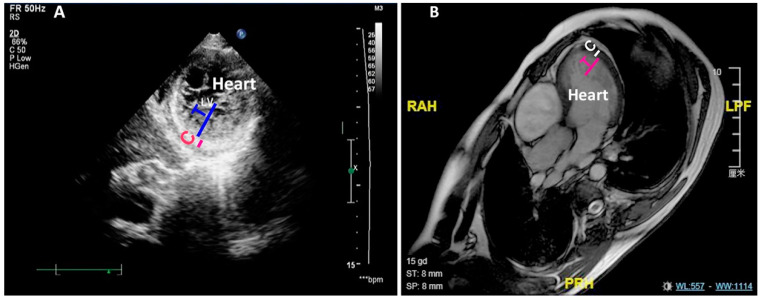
LVNC is detected by ECHO and MRI. (A) Shows the noncompaction measured by ECHO of an LVNC patient. (B) Shows the noncompaction measured by MRI of an LVNC patient. C: compact zone; T: trabecular zone; LV: left ventricle; ECHO: echocardiography; MRI: magnetic resonance imaging.
